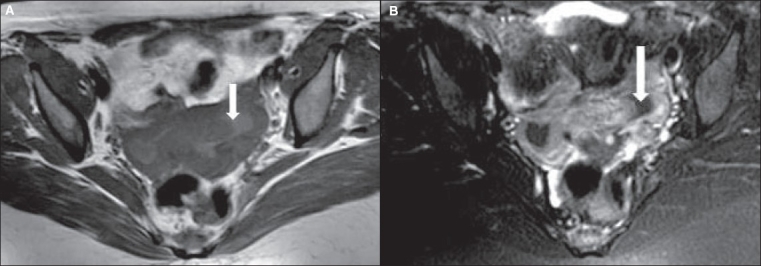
Axial MRI of the uterus shows the contents of the mass to be of high signal intensity on T1W image (A) and of low signal intensity on T2W image (B), consistent with blood products
